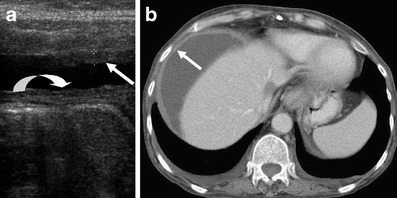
Peritoneal thickening seen on ultrasound (a) of the abdomen demonstrates parietal (*straight arrow*) and visceral (*curved arrow*) peritoneal thickening separated by a small amount of free fluid. Axial IV contrast enhanced CT (b) portal venous phase in the same patient demonstrates parietal peritoneal thickening (*arrow*) in the upper abdomen
